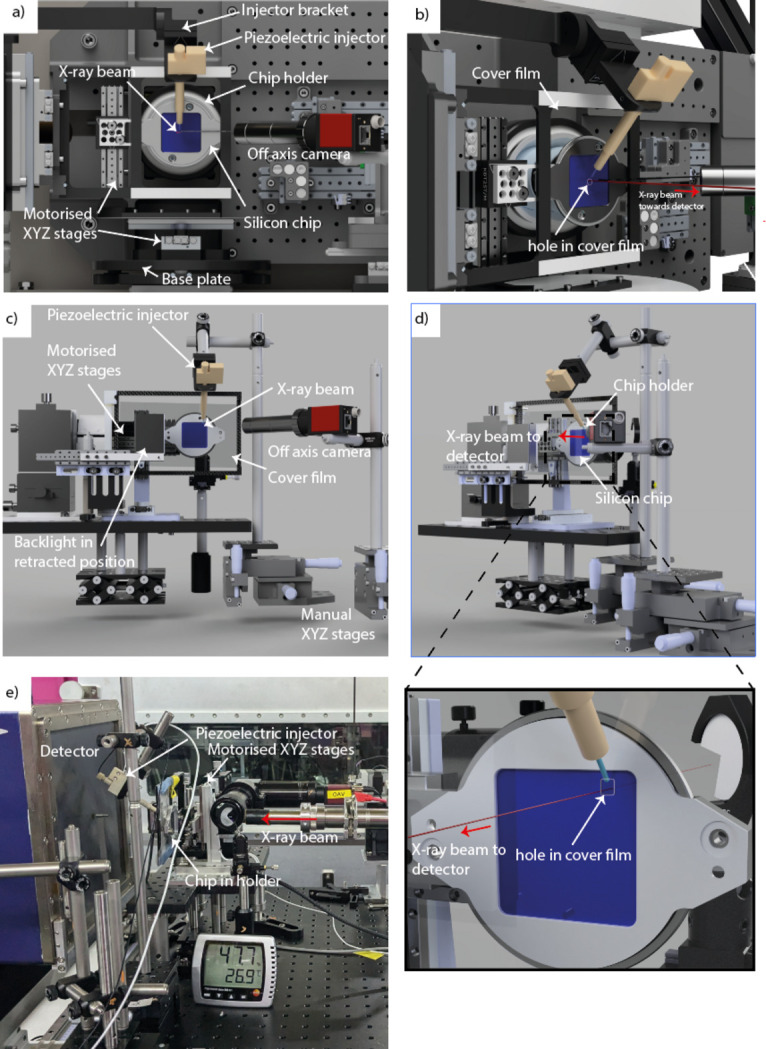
Drop-on-fixed-target setup at various endstations. A typical setup consists of a fixed-target chip, containing an open face, which is covered by a cover film held in place by a frame. An off-axis camera is used to align the droplet ejection with the wells. (*a*) Rendered view of the drop-on-fixed-target setup at I24 Diamond Light Source (DLS) observed from the detector position looking upstream into the X-ray beam; (*b*) rendered view of the drop-on-fixed-target setup at I24 DLS from the side, highlighting the hole in the cover film through which droplets can be added to the fixed-target chip; (*c*) rendered view of the drop-on-fixed-target setup at the NCI endstation at PAL-XFEL; (*d*) rendered view from the side of the setup at the NCI endstation at PAL-XFEL; (*e*) photograph of the drop-on-fixed-target setup at the NCI endstation at PAL-XFEL.
